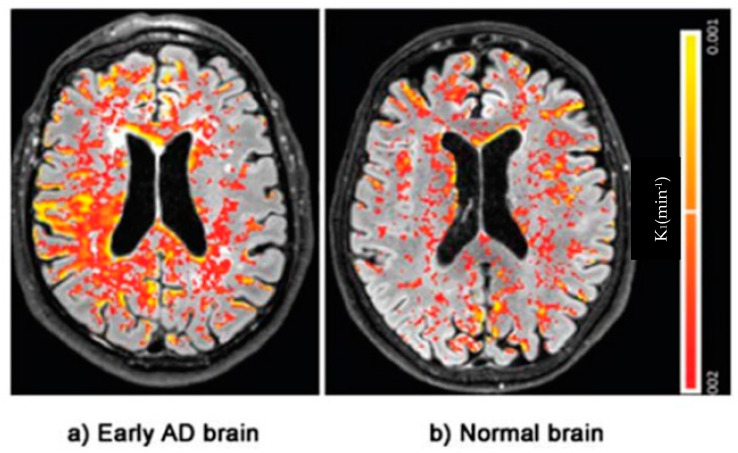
DCE Magnetic resonance brain image of an Alzheimer’s patient with color-coded (Red–Yellow) blood–brain barrier leakage. Note the greater extent of leakage in AD. Adapted from Evidence of BBB damage in the AD brain: (a) extensive leakage of gadobutrol (an MRI contrasting agent) through a damaged BBB in brains of patients with early signs of AD; (b) less extensive leakage of the agent in brains of normal patients. Adapted with permission from [77], published by the Radiological Society of North America, Inc., 2016.
